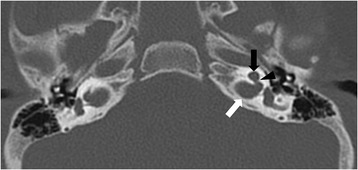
Computed tomography image of the temporal bone of a patient with HDR mimicking a congenital X-linked stapes gusher syndrome. Axial CT section of the temporal bones shows severe cochlear hypoplasia (*black arrow*), bulbous dilatation of the fundi of the IAC (white arrow) and a fistulous connection between the internal auditory canal and the cochlear basal turn with absence of the bony partitions (*black arrowhead*) on the left. These findings were seen on the right side (not shown) IAC; Internal Auditory Canal
